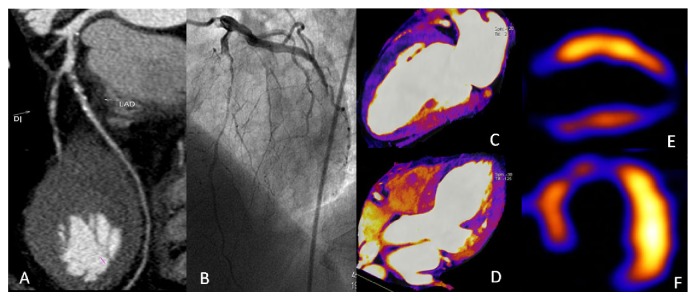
Curved multiplanar reformation of CCTA (A) shows severe stenosis in the left anterior descending artery (LAD). Coronary angiography (B) demonstrates the occlusion in the proximal LAD. DE-CTP images show hypoperfusion at the anterior, apical, septal, and inferior walls in the two and four-chamber views (C and D). SPECT-MPI images reveal perfusion defects in the same regions (E and F).
